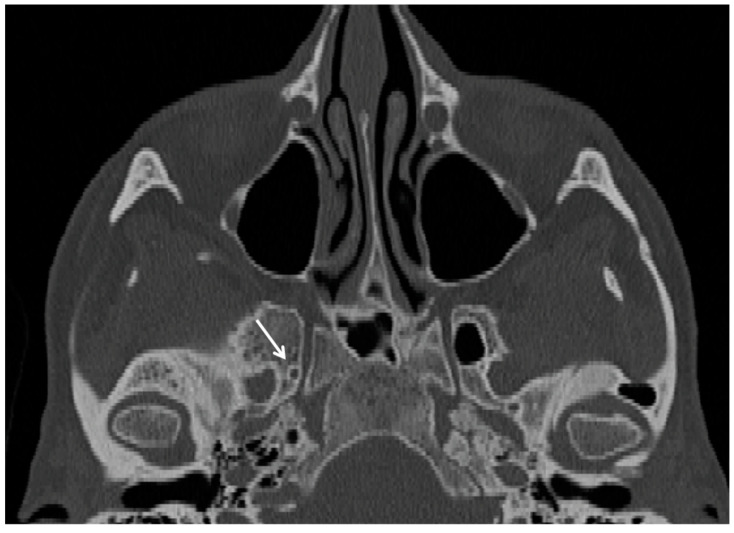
Tranverse CT scan of an individual with the right foramen of Vesalius (white arrow).
